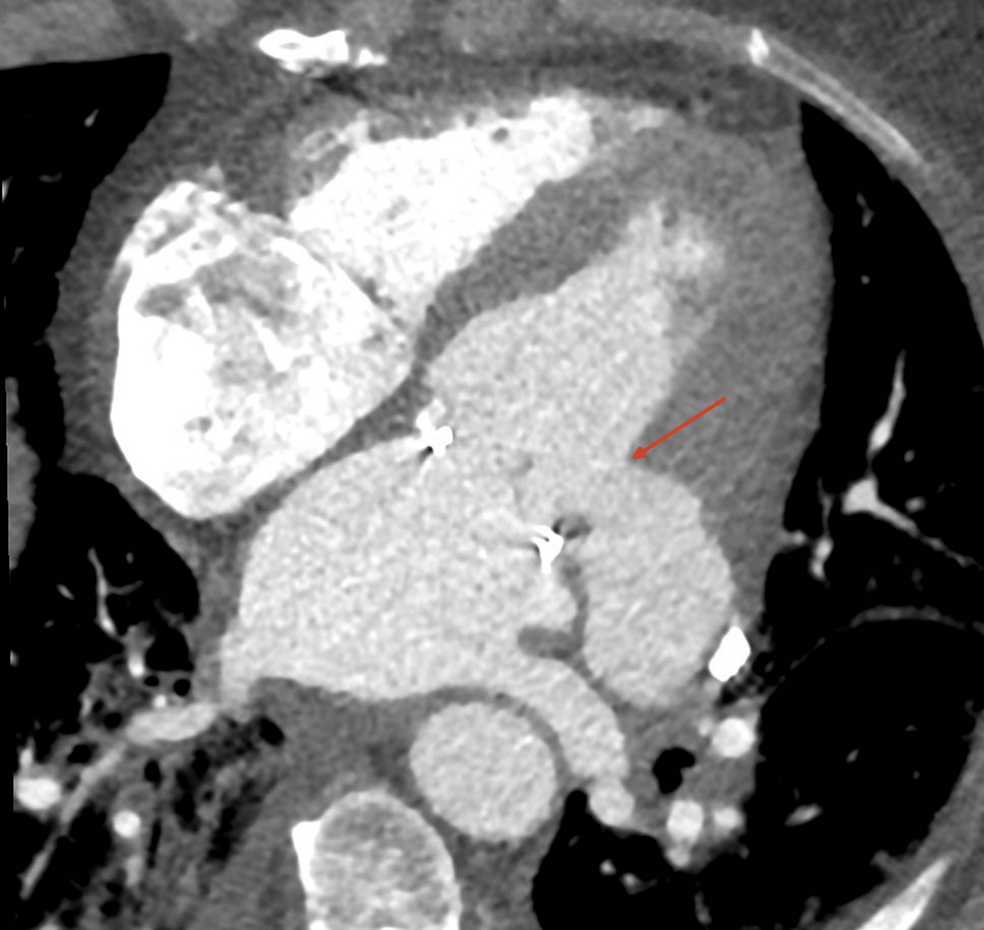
Cardiac computed tomography in a four-chamber view. The red arrow indicates the neck of the left ventricular pseudoaneurysm distal to the mitral valve prosthesis.
